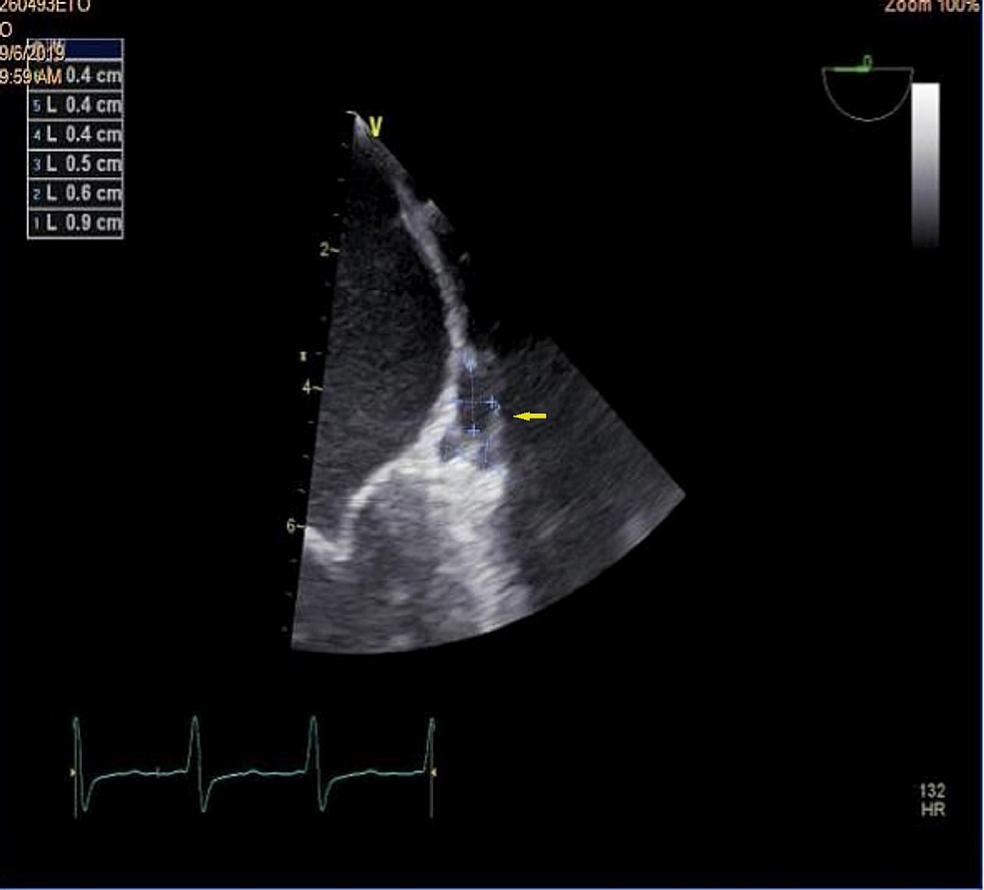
Presence of three abscesses in the aortic ring on echocardiography.
